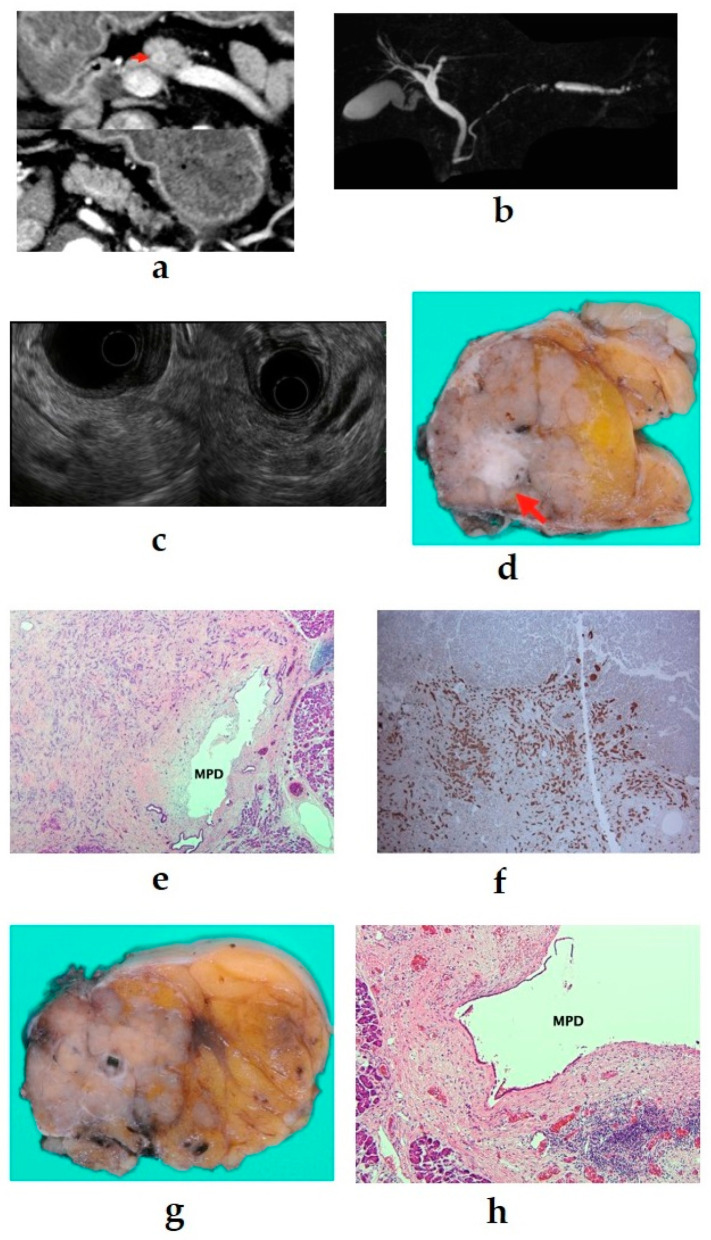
(a) Contrast-enhanced computed tomography (CT): a mass lesion with early hyperenhancement is seen in the pancreatic body (red arrow), but there are no other obvious lesions. (b) Magnetic resonance imaging: the main pancreatic duct (MPD) in the pancreatic body is extensively narrowed, and the caudal duct is dilated. (c) Endoscopic ultrasound (EUS): a circular hypoechoic mass in the pancreatic body. Pancreatic duct stenosis is observed even in the absence of mass. (d–f) 6-mm neuroendocrine neoplasm G1 (red arrow), serotonin positive, with stromal fibrosis (40×). (g,h) Pancreatic duct stenosis due to stromal fibrosis upstream of the tumor (40×).
